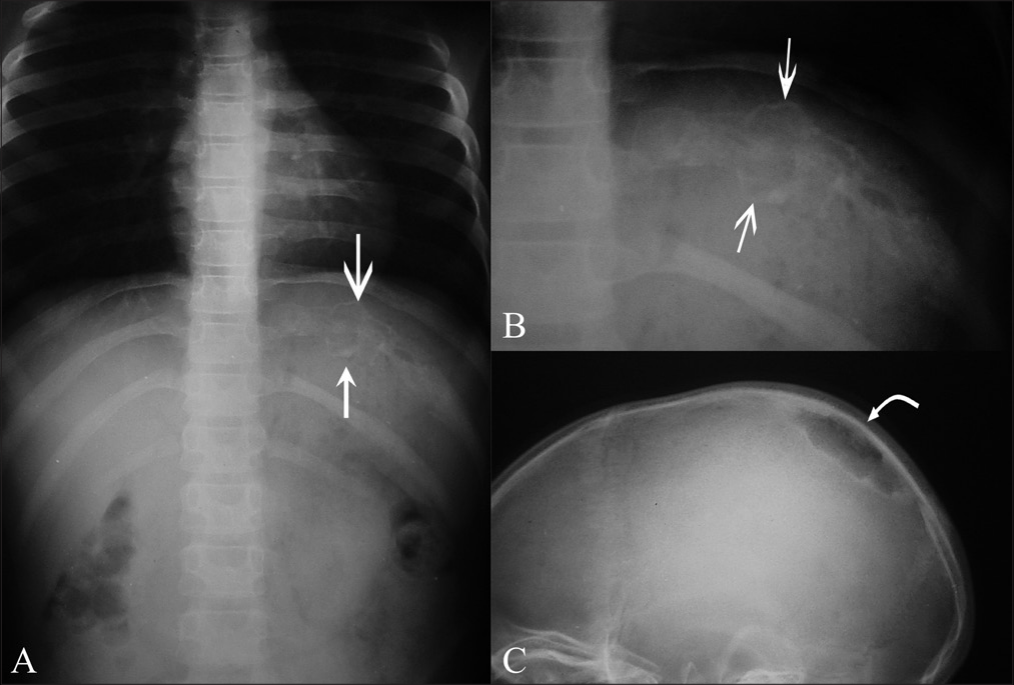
Rib tuberculosis – patient 8. Frontal radiographs of the ribs (A, B) show an expansile osteolytic lesion with sclerosis involving the posterior end of the left 10^th^ rib (arrows). Lateral skull radiograph (C) of the same patient shows a sharply marginated osteolytic lesion, with marginal sclerosis in the right parietal bone (curved arrow)
